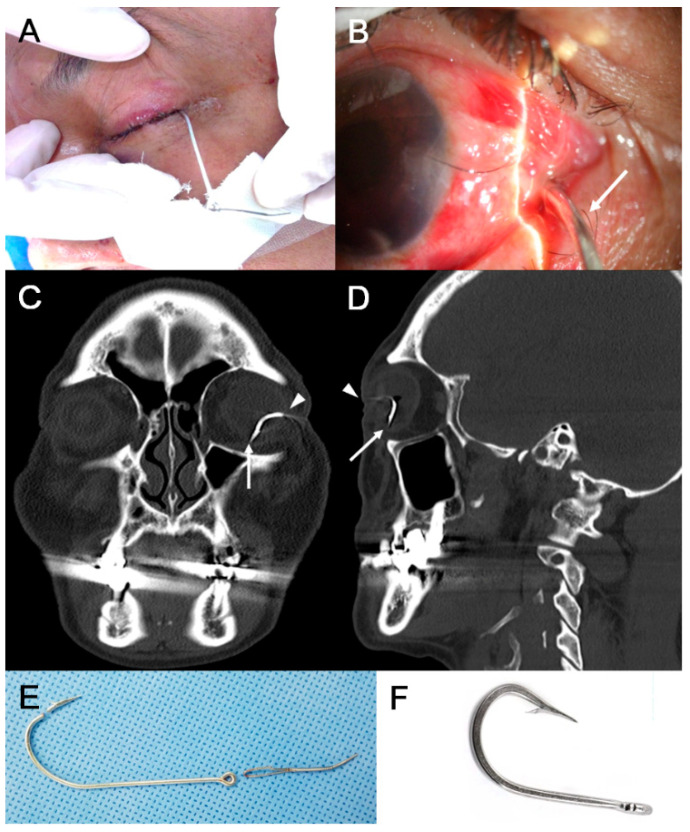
Anterior segment photograph and CT scan of a 54-year-old male patient injured by a fishhook while pulling it during fishing. (A,B) The fishhook (*long white arrows*) has penetrated the temporal sclera in the left eye, and the distal end of the needle is not identified. (C,D) Coronal and sagittal CT images of the patient showing the fishhook penetrating the eyeball (*white arrowheads*) and the double perforation into the attachment site of the inferior rectus muscle (*long white arrows*). (E) Since the fishhook cannot retrieved through the inlet due to the barb, it is moved forward, and the lower part of the barb is cut with a wire cutter. The remaining fishhook is removed by moving backward toward the inlet. (F) The removed fishhook is a simple single-barbed fishhook.
